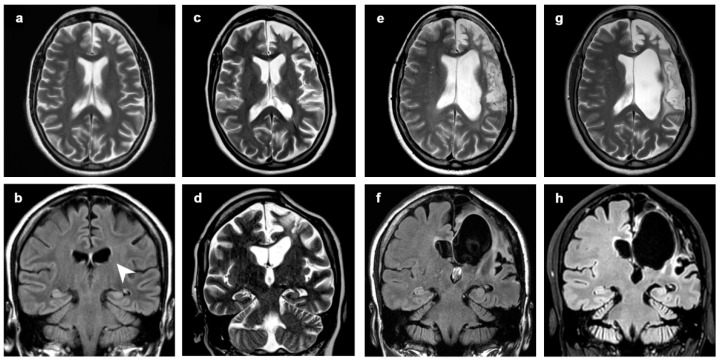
Patient 3, progression of the disease in 17 years. Axial (a,c,e,g) and coronal (d) T2-weighted images, coronal FLAIR (b,f,h) at (a,b) 2 years, (c,d) 5 years, (e,f) 10 years and (g,h) 17 years from clinical onset. In the first MRI (a,b), only subtle dilatation of left ventricle (arrowhead) and enlargement of frontal sulci could be seen. In (d), WM hyperintensity preceded the later atrophic change. Surgical treatment was performed at 6 years from onset, between (c,d) and (e,f); after surgery, EPC was controlled. At last follow-up, seizures and cognitive and behavioral disorders were present.
